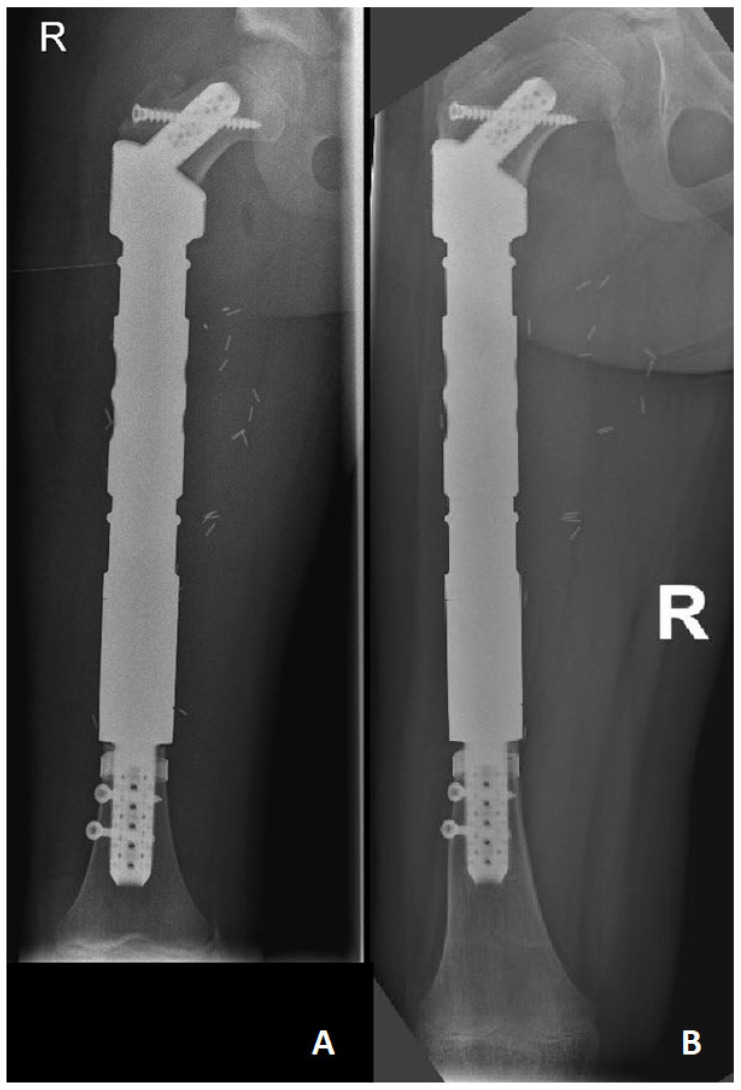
Due to Ewing’s sarcoma located in the right femur diaphysis (case no 6), an intercalary prosthesis was applied using a hollow stem specially prepared for the patient’s defect in the proximal and distal parts. (A) Post-operative X-ray of the right femur (a. p.) (B) X-ray (a. p.) at 36-month follow-up with no sign of implant failure, stem loosening, or local recurrence.
